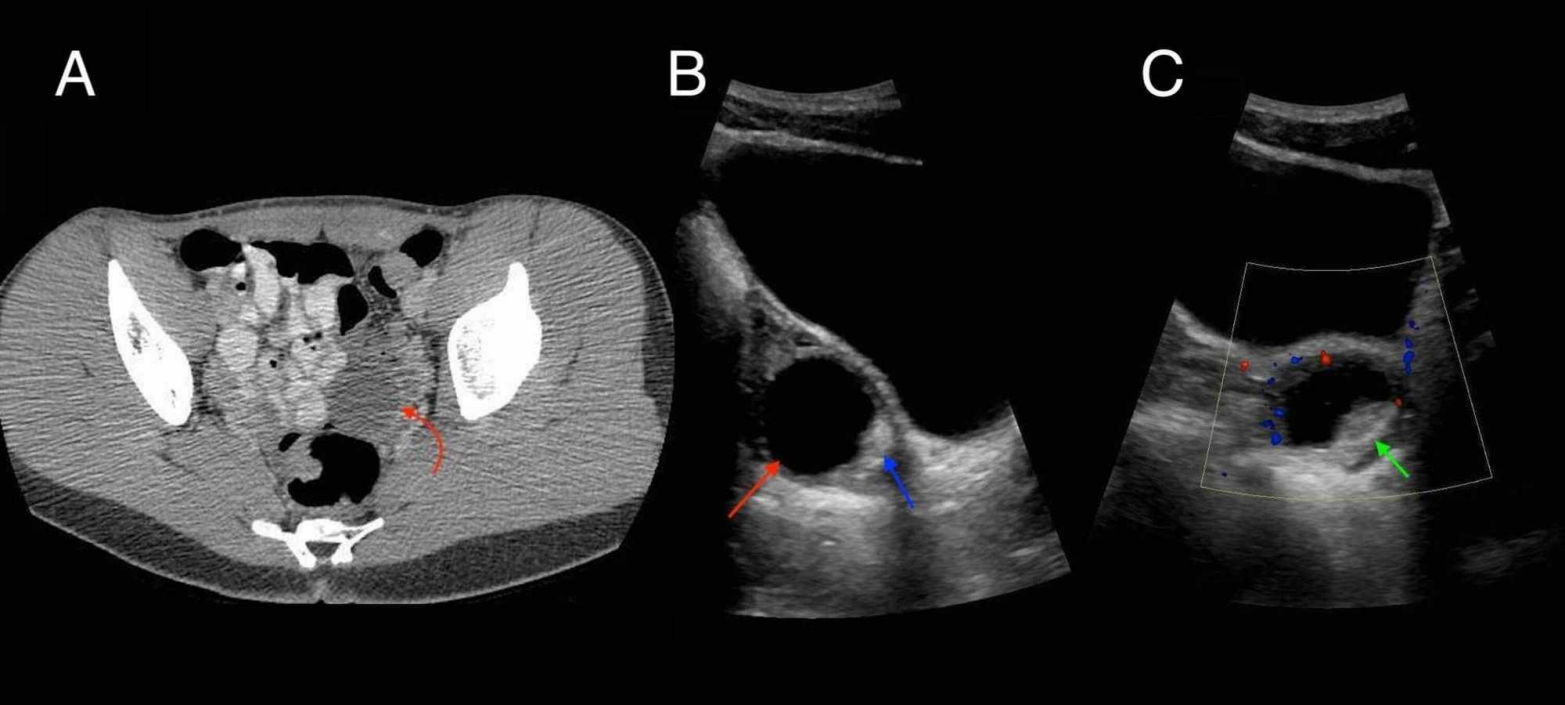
CT of abdomen and pelvis and US of pelvis. (A) Axial CT image of the abdomen and pelvis demonstrates a 3.5 cm left adnexal mass (curved red arrow) which measures fluid density centrally (approximately 4 houndsfield units). It is located anterior to the rectum and adjacent to loops of contrast-filled small bowel. (B) Sagittal ultrasound image of the pelvis without Doppler interrogation demonstrates a 3.5 cm anechoic cystic lesion within the left ovary (red arrow). The lesion demonstrates posterior acoustic enhancement. There is a smaller hyperechoic nodule along the posteroinferior margin of the lesion (blue arrow). (C) There is no definite blood flow within this nodular focus on the sagittal Doppler image (green arrow) US, ultrasound
